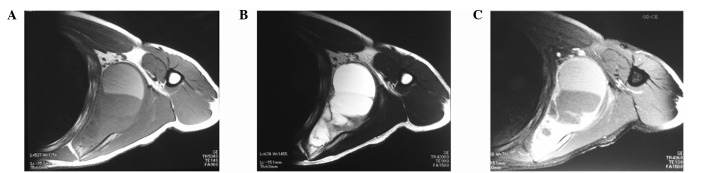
(A) Axial T1-weighted (TR/TE, 534/14) spin echo image of the left axilla showed a cystic tumor with fluid-fluid level, indicative of a soft tissue sarcoma. (B) Axial T2-weighted (TR/TE, 4200/99) spin echo image showed a large cystic tumor. (C) Gadopentetic acid heterogeneously enhanced the mass (TR/TE, 436/13). TR/TE; repetition time/echo time.
